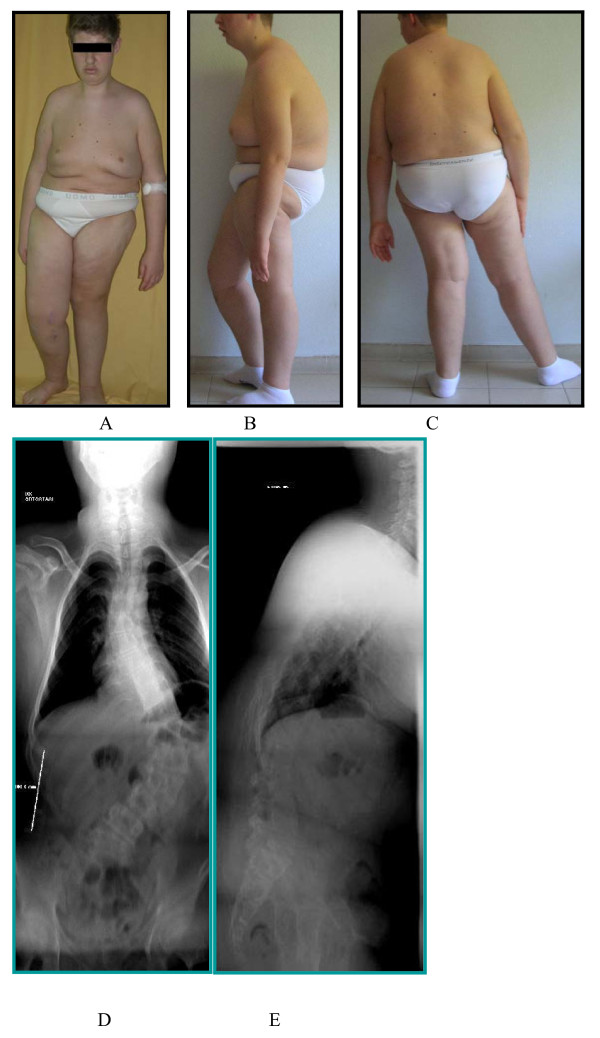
Preoperative clinical and radiographic picture. O.M., male, born in 1992, operated on in 2007; severe kyphoscoliosis in PWS in a patient affected with valgus knee deformity.
